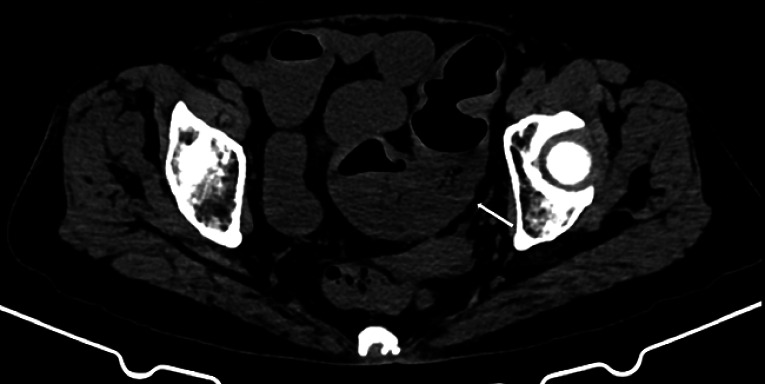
Abdominal computed tomography in an elderly woman, showing a sigmoid colon mass with distension of the distal bowel (arrow).
